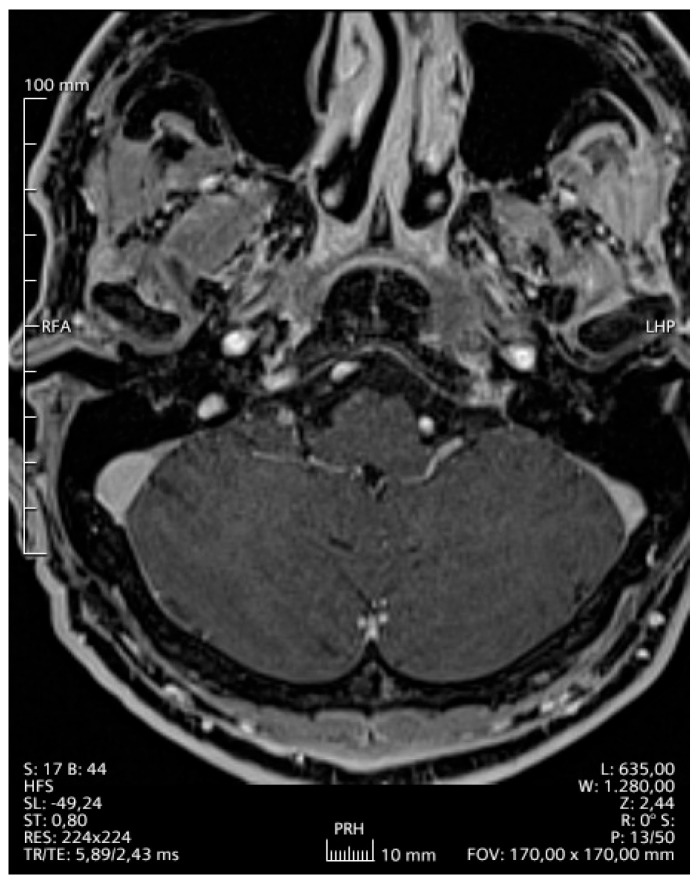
Second MRI 10 months after the first MRI and 8 months after initiation of the treatment against *Mycobacterium xenopi,* (T1 sequence gadolinium-enhanced) showing no gadolinium-enhancing lesions.
